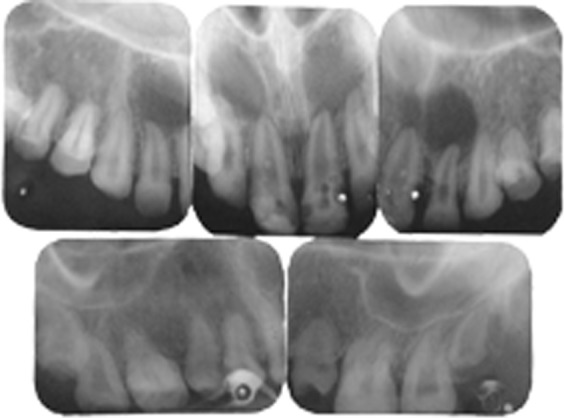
IOPAR of dentition in upper arch showing periapical radiolucency in relation to upper right and left central and lateral incisors
